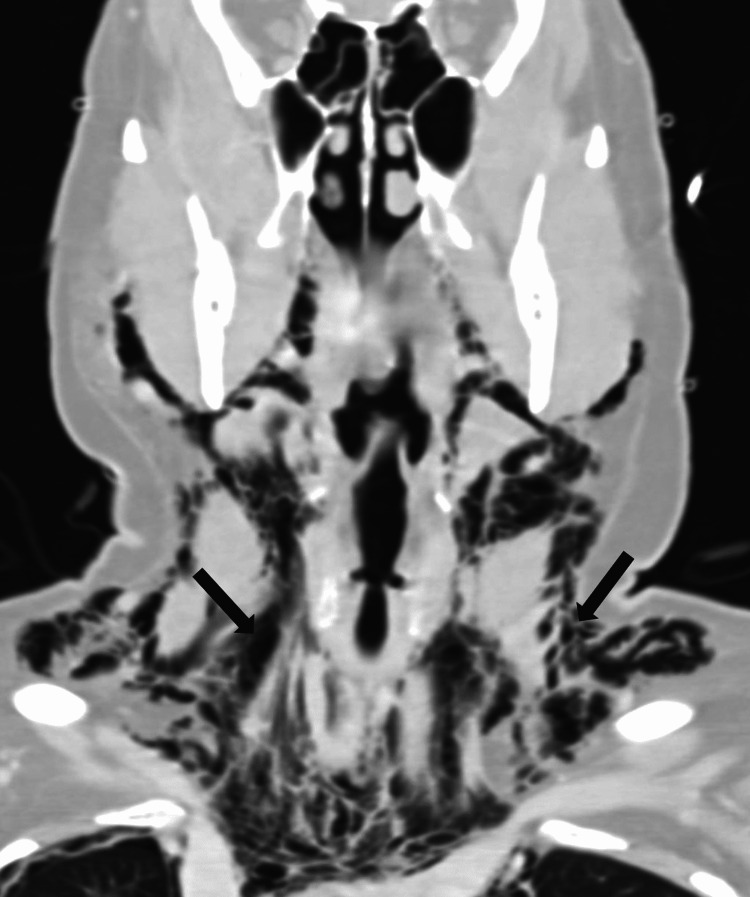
Neck CT showing extensive subcutaneous emphysema in the soft tissues of the neck
